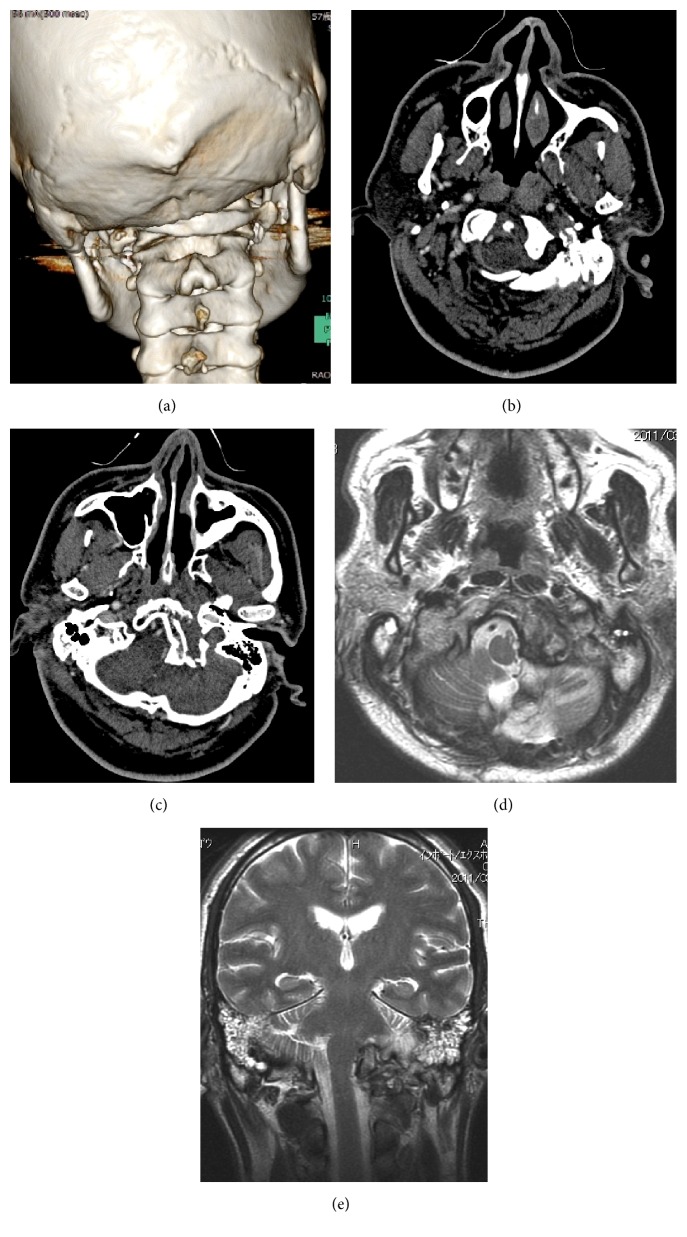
Images from a three-dimensional computed tomography (a), enhanced computed tomography (b and c), and magnetic resonance imaging (d and e) when the patient was first examined. The dislocated C1 that accompanied the occipital condyle fractures was intracranially impacted (a, b, and c). The left vertebral artery was not revealed by CT angiography (b and c). Cerebellar edema occurred due to traumatic hemorrhagic cerebellar contusions, which compresses the brainstem (d and e).
